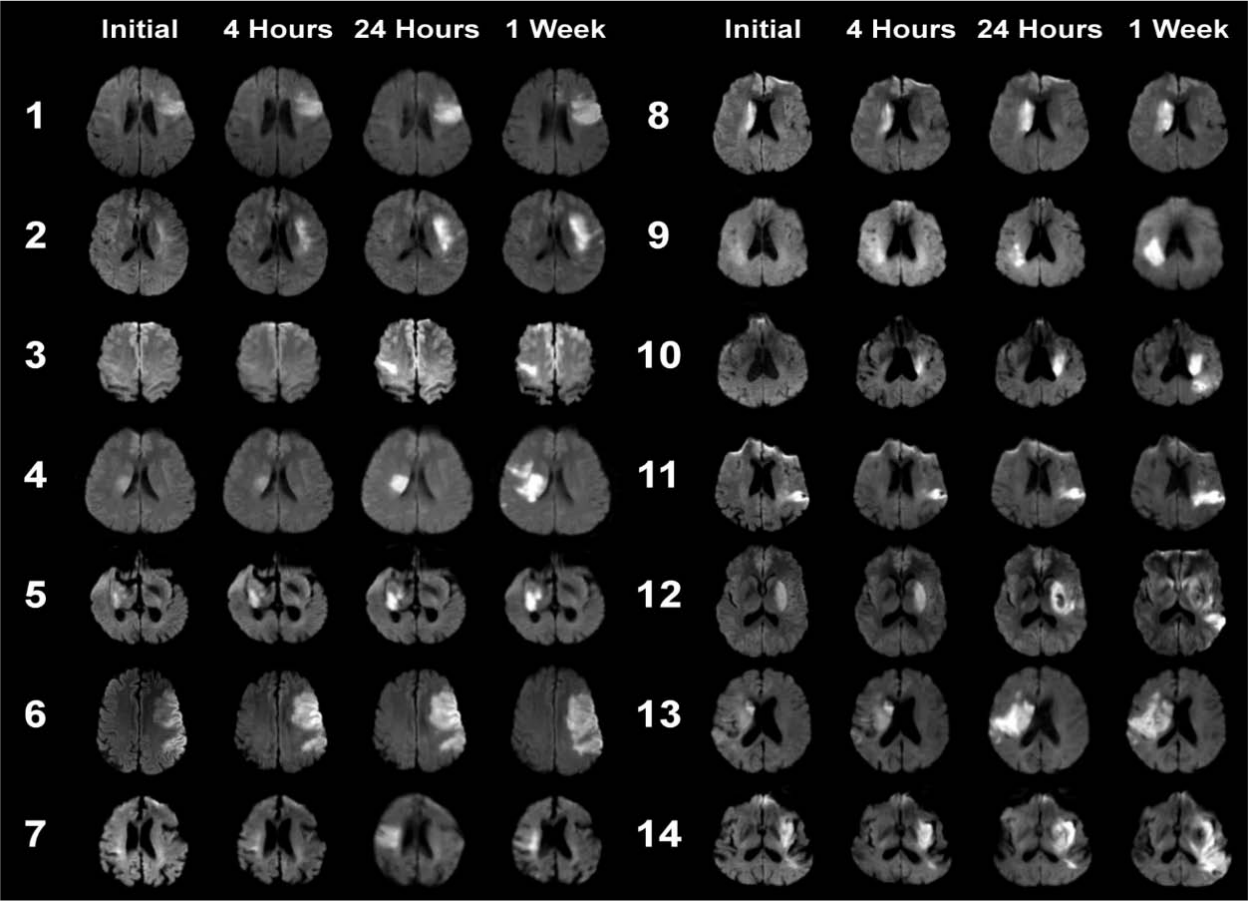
Diffusion weighted images from all 14 patients. Images were selected at the level of maximum lesion growth for each patient. The baseline, 4 hour, 24 hour and 1 week time points are displayed. The DWI hyperintense abnormalities did not change in most patients at 4 hours after baseline. However, there was an obvious increase in lesion volume by 24 hours after baseline in most patients, as well as at the 1 week time point.
